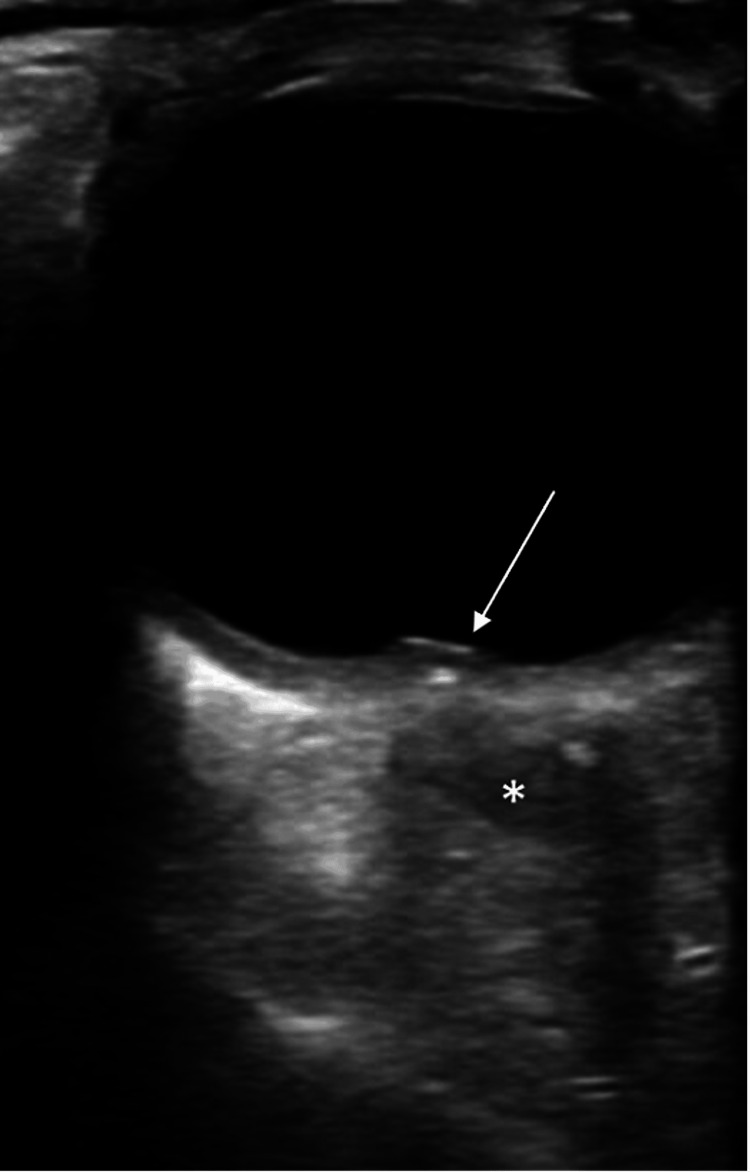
Point of care ocular ultrasound Ocular ultrasound demonstrating optic disc elevation (arrow) with a dilated, hypoechoic optic nerve sheath (*) just posteriorly, suggestive of increased intracranial pressure and papilledema
